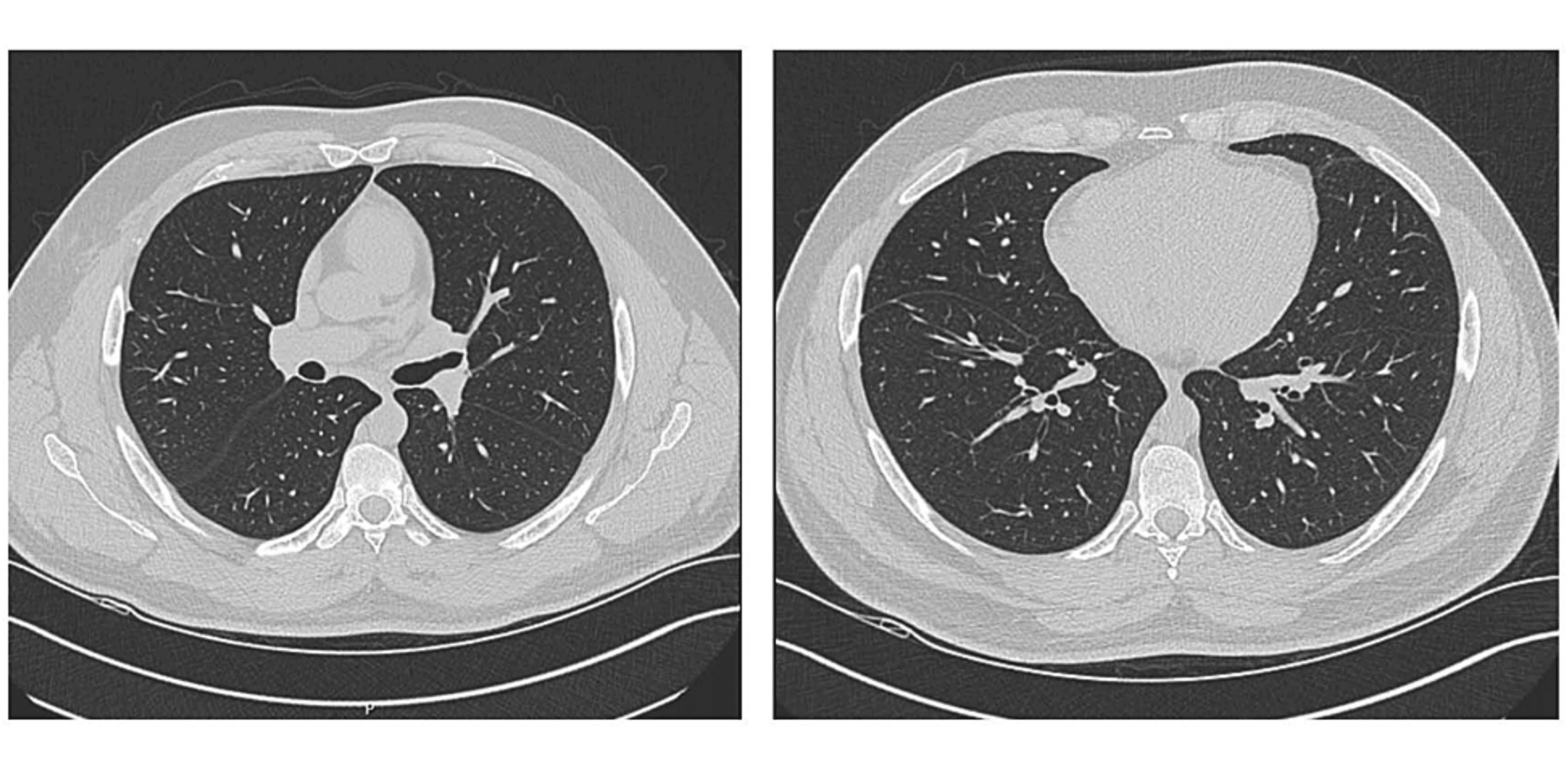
High-resolution CT chest demonstrating resolution of hypersensitivity pneumonitis (HP).
